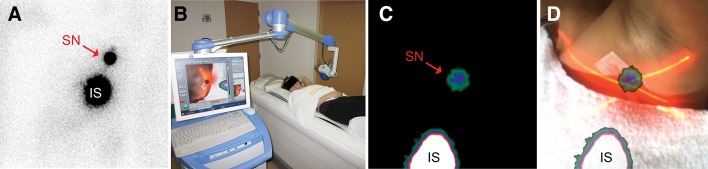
Comparison of preoperative images. In a patient with breast cancer, oblique planar lymphoscintigraphy (a) 2 h post-injection showed one sentinel node (SN) in the left axilla. The portable hybrid camera was placed above the lymphatic field to obtain an overview image at a distance of approximately 15 cm (b). Standard portable gamma camera imaging showed the injection site (IS) and SN in the same relation to each other, but without any anatomical references (c). Combined optical and γ-imaging visualised the image field of view and anatomical SN location in the left axilla (d). In the fused image, the SN is visualised on *top* of the *red* laser pointer cross indicating an accurate image fusion (Figure reproduced with permission from [21]—Hellingman et al. A new portable hybrid camera for fused optical and scintigraphic imaging: first clinical experiences. Clinical Nuclear Medicine (http://journals.lww.com/nuclearmed), 41(1):e39–e43, 2016. Promotional and commercial use of the material in print, digital or mobile device format is prohibited without the permission from the publisher Wolters Kluwer. Please contact healthpermissions@wolterskluwer.com for further information)
